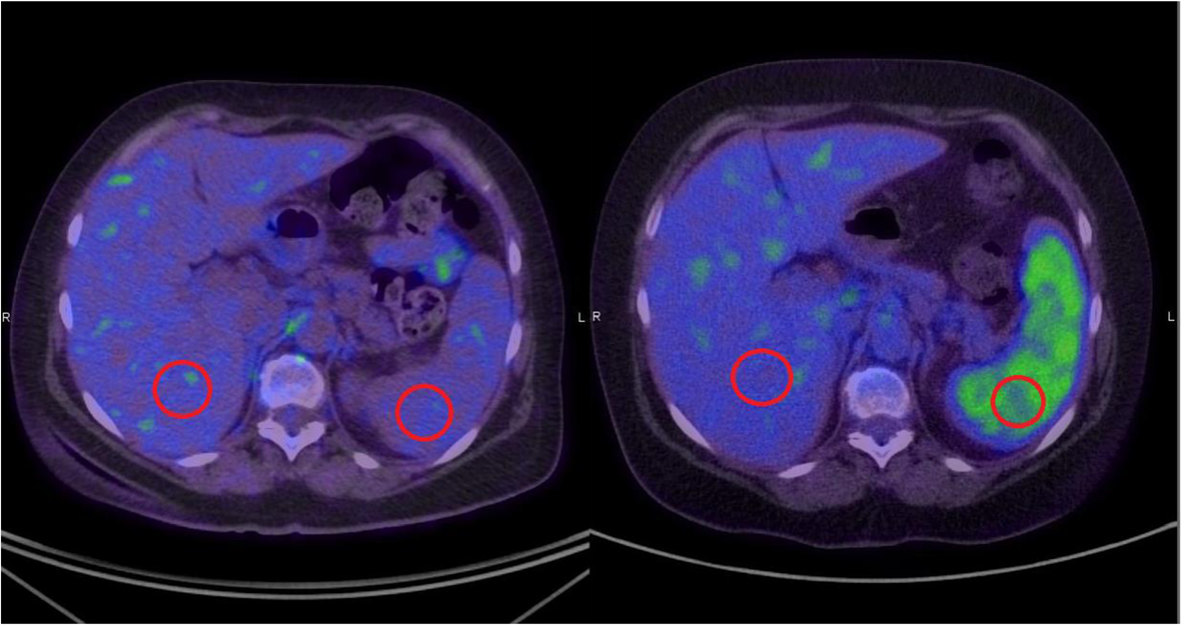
Spleen to Liver Ratio (SLR) on positron emission tomography. Example of normal splenic FDG tracer uptake (left) compared to abnormally high tracer uptake within the spleen (right). The SUV is calculated by placing 2 cm spherical volumes of interest in the liver and spleen respectively (as depicted by red circles). The spleen to liver ratio (SLR) is calculated by dividing the SUVmean of the spleen by the SUVmean of the liver. SLR > 1.1 is considered abnormally high
